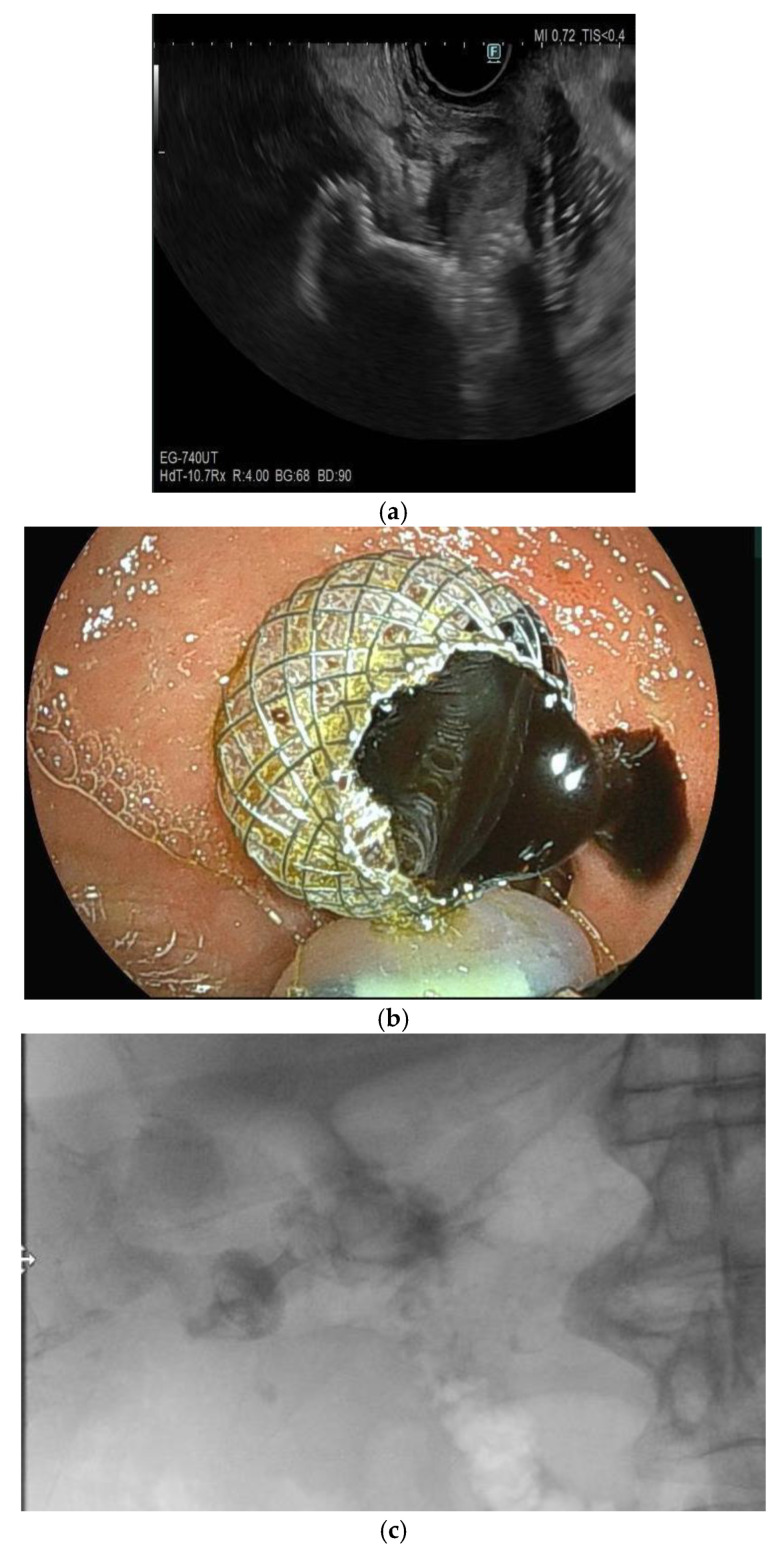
(a) Endoscopic ultrasound image of gallbladder drainage with LAMS, with distal flange opened correctly inside the gallbladder and proximal flange in the GI lumen. (b) Endoscopic image of proximal flange open in the GI lumen with bile flow. (c) Fluoroscopy image of LAMS deployment in correct position.
